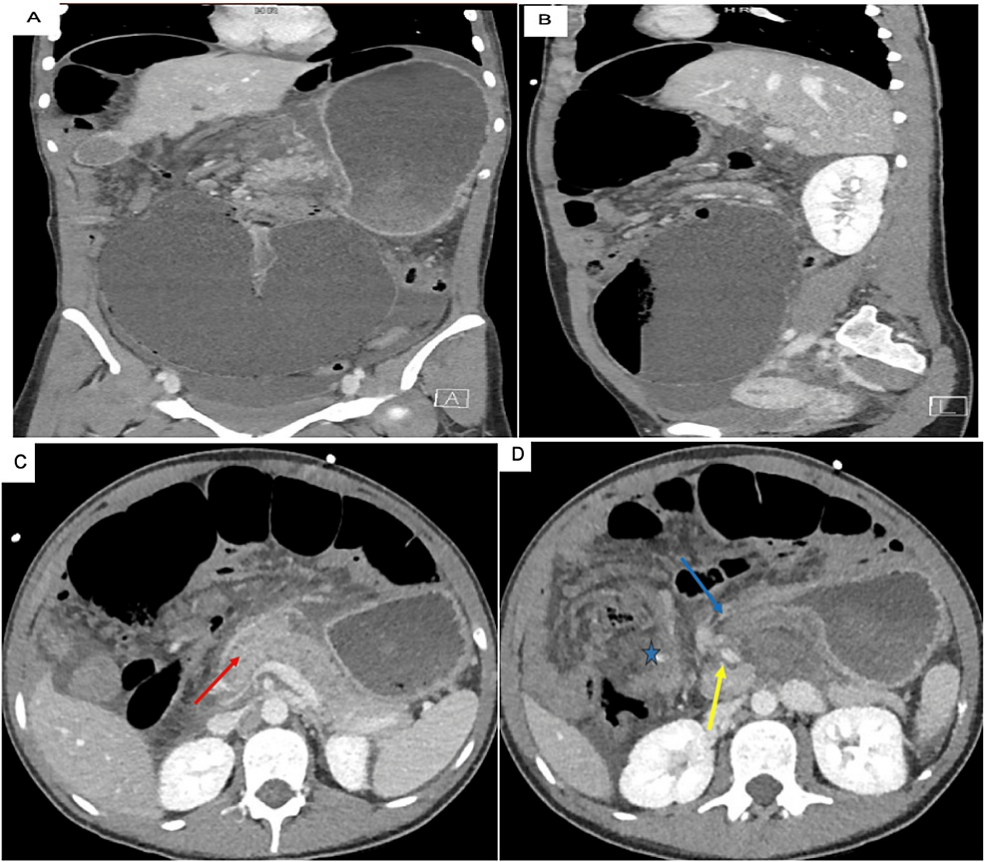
(A) Coronal and (B) sagittal views demonstrating megaduodenum. (C) and (D) Axial views demonstrating duodenal volvulus. A) Coronal and B) sagittal computed tomography images demonstrating megaduodenum extending into the pelvis and closed-loop obstruction from the duodenal volvulus with pneumatosis intestinalis concerning for ischemia. C) Axial view of the duodenal volvulus with a significant mesenteric swirling with the pancreas (red arrow) being pulled into the volvulus. D) Axial view showing the volvulus with the distal stomach (blue arrow) and superior mesenteric artery (SMA) (yellow arrow) being pulled to the right of the midline by the volvulus. The blue star indicates a dilated duodenum with surrounding mesenteric swirling.
